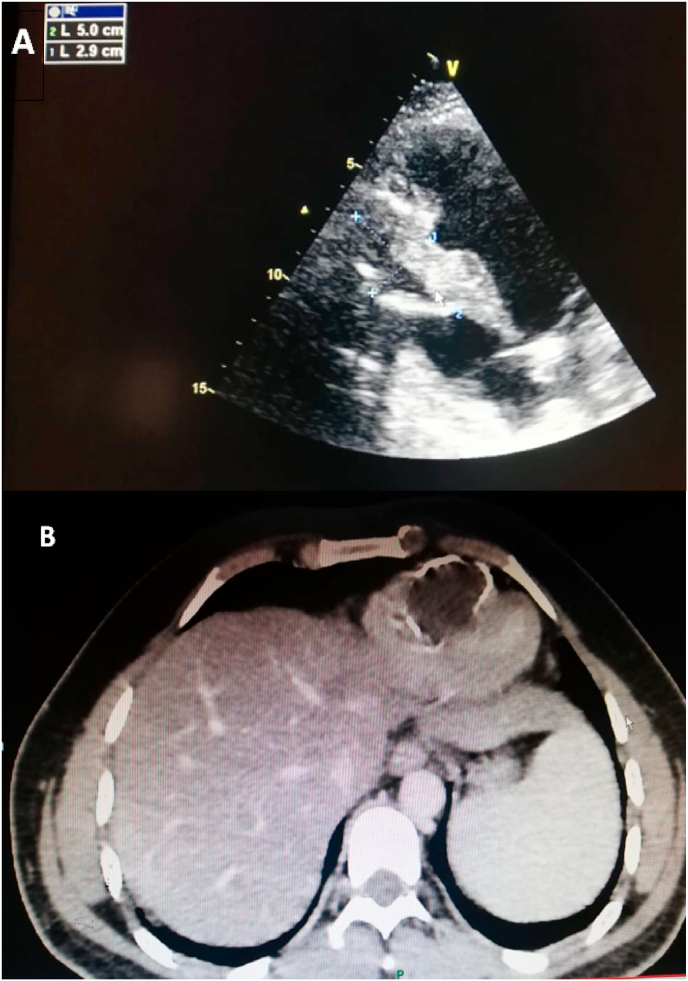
(A; B) Transthoracic echocardiography and Chest computed tomography imaging showing a right ventricular hydatid cyst.
